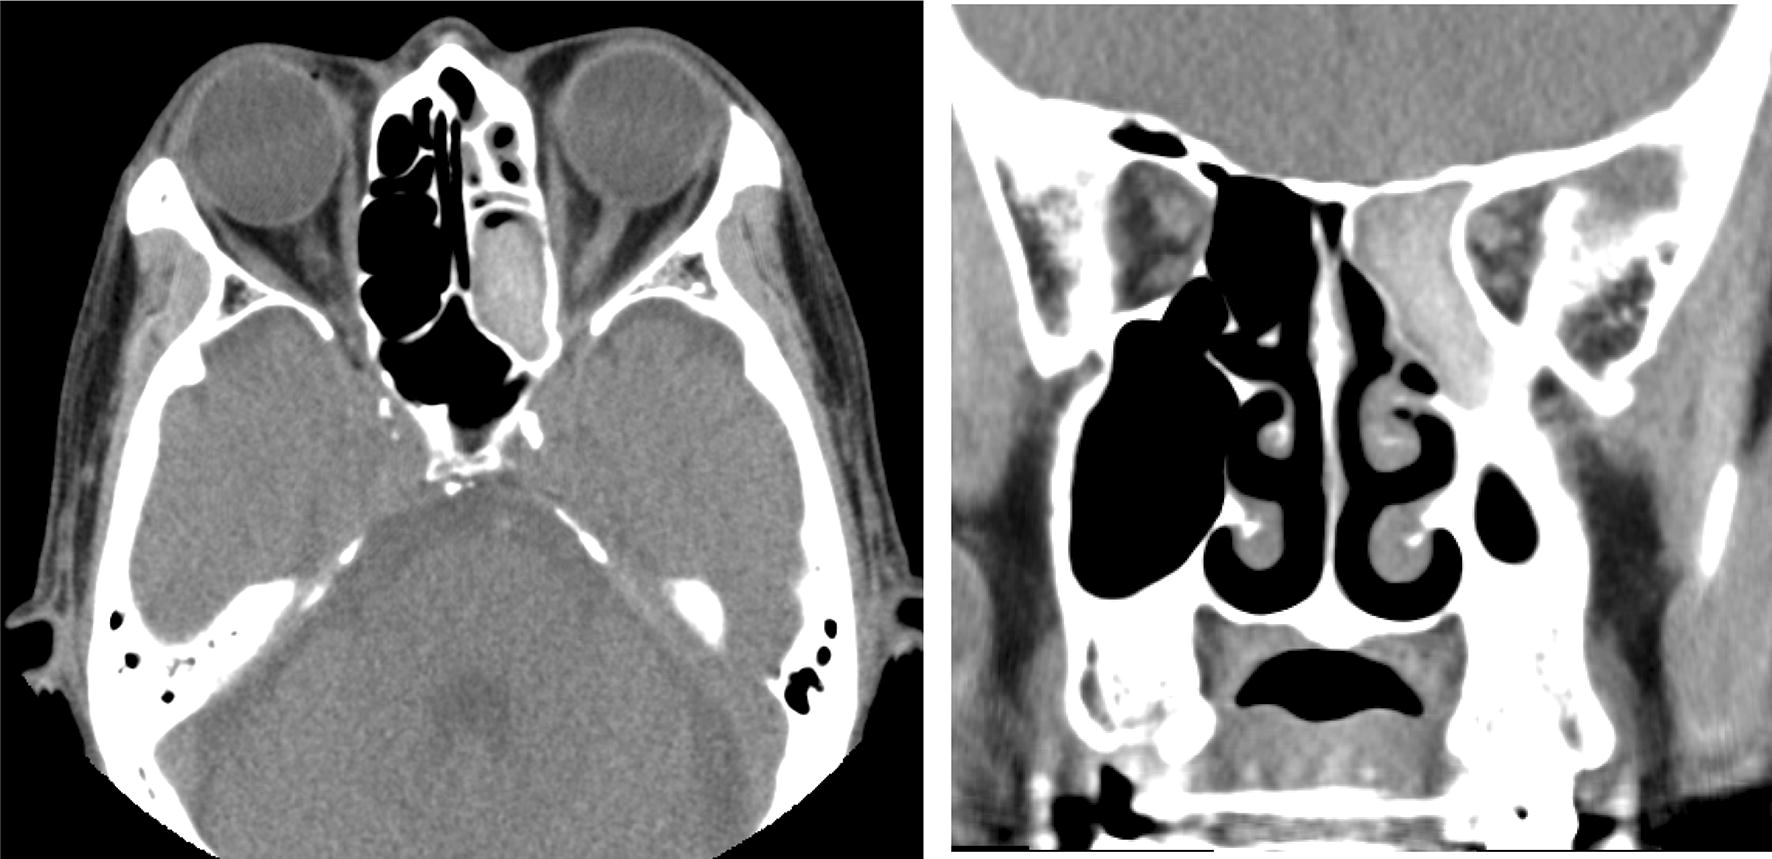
Computed tomography of paranasal sinuses revealing high-attenuation mucus plug in the left ethmoid sinus similar to that observed in the right intermediate bronchial trunk
